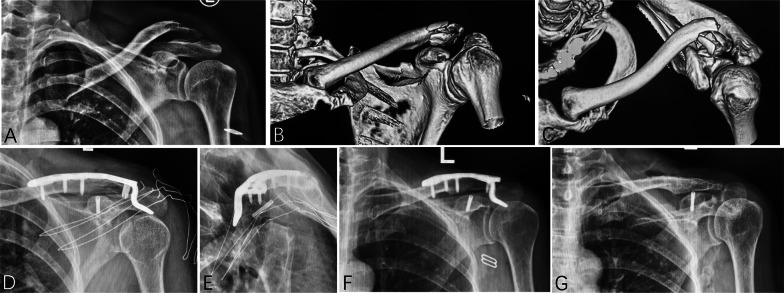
A 56-year-old woman (patient 3) was injured by a car while crossing the road. Preoperative A X-ray and B, C computed tomography (CT) scan showed a displaced comminuted distal clavicle fracture combined with ipsilateral Eyres type IV coracoid process fracture. D, E The distal clavicle fracture was fixed with a clavicle hook plate, and the coracoid process was fixed with a headless screw. F X-ray taken 20 months postoperatively showed solid bone union with no implant failure, and the patient was very satisfied with the treatment and returned to her pre-injury work. G She later underwent implant removal
